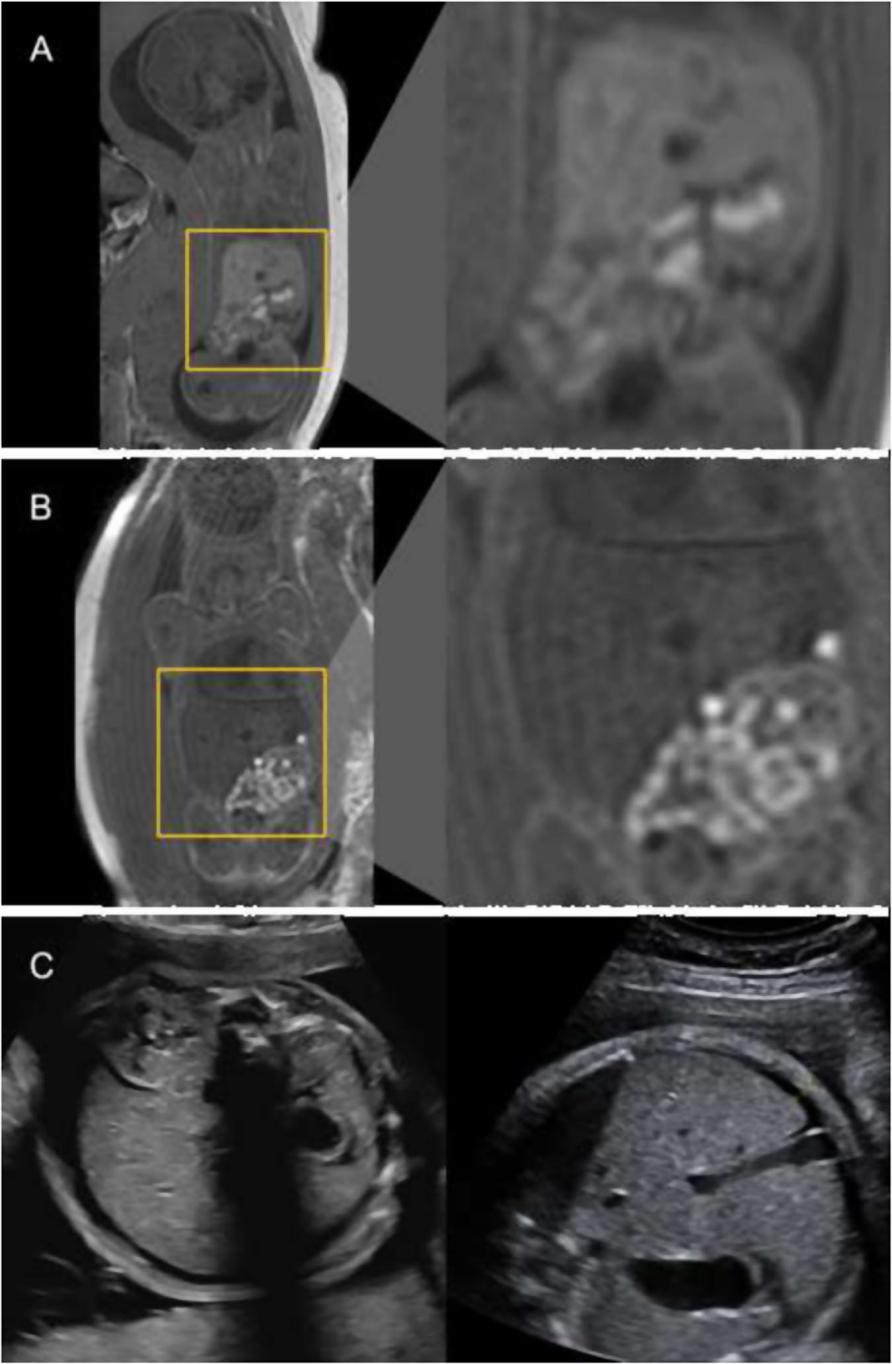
Effects of intrauterine transfusions on fetal liver parenchyma on T1-weighted magnetic resonance (A, B) and ultrasound imaging (C). A Picture A shows a fetus at a gestational age of 28 + 4 weeks, who did not receive IUT. On T1-weighted sequences, the liver parenchyma imposes hyperintensely (signal intensity (SI) = 458) compared to surrounding tissues. B Images of a different fetus at a gestational age of 28 + 5 weeks, who received a total of two IUTs, the last one 24 days prior to MRI acquisition. Compared to the fetus who did not receive IUT, the liver parenchyma appears darker (SI = 304) on T1-weighted sequences, even though the procedure was performed several weeks prior. C For comparison, ultrasound images of a third fetus (examinations from gestational age 29 + 0 and 30 + 0) are shown. The fetus received a total of five IUTs during pregnancy, one between the two ultrasound examinations. No apparent effects on parenchyma echogenicity were detectable. The thin lamella of ascitic fluid was most likely due to the known parvovirus B19 infection
